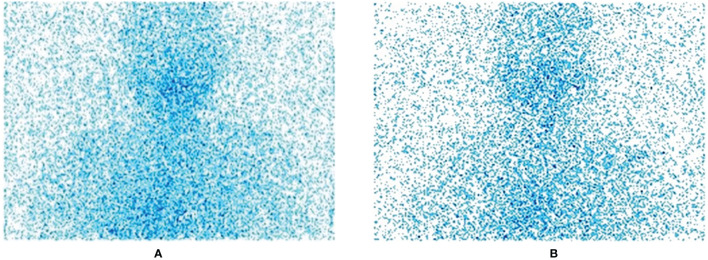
(A) the original SPECT image with residue; (B) the original SPECT image without residue.
